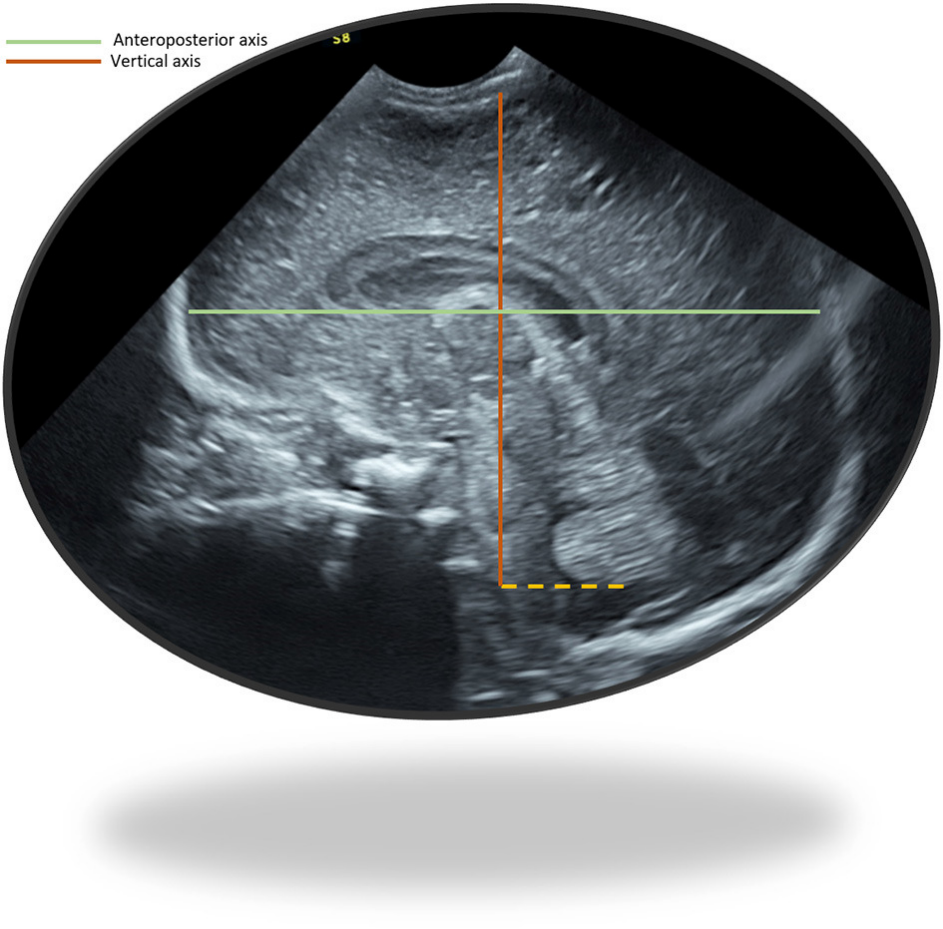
Anteroposterior axis and vertical axis measured in an ultrasonographic sagittal plane.
